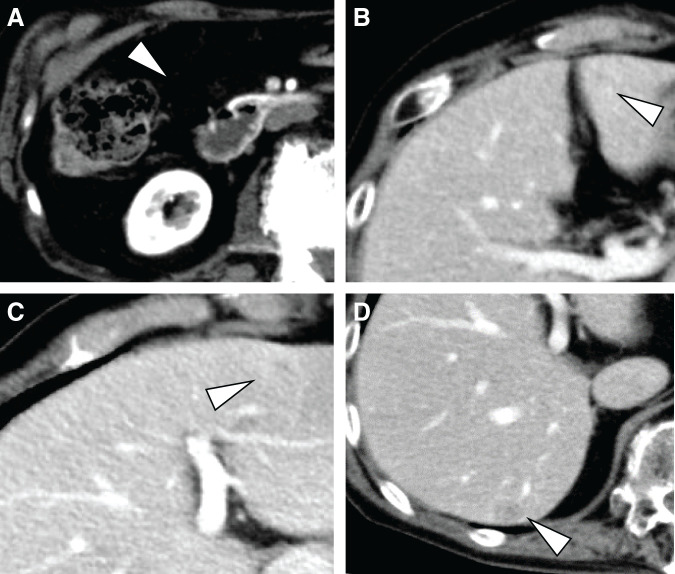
Tumor response to sunitinib and disease progression. (A–C) Abdominal CT of lesions that responded to sunitinib (arrowhead). (D) An abdominal contrast-enhanced CT scan revealed an increase in the size of a lesion in liver segment 7 (S7) (arrowhead).
